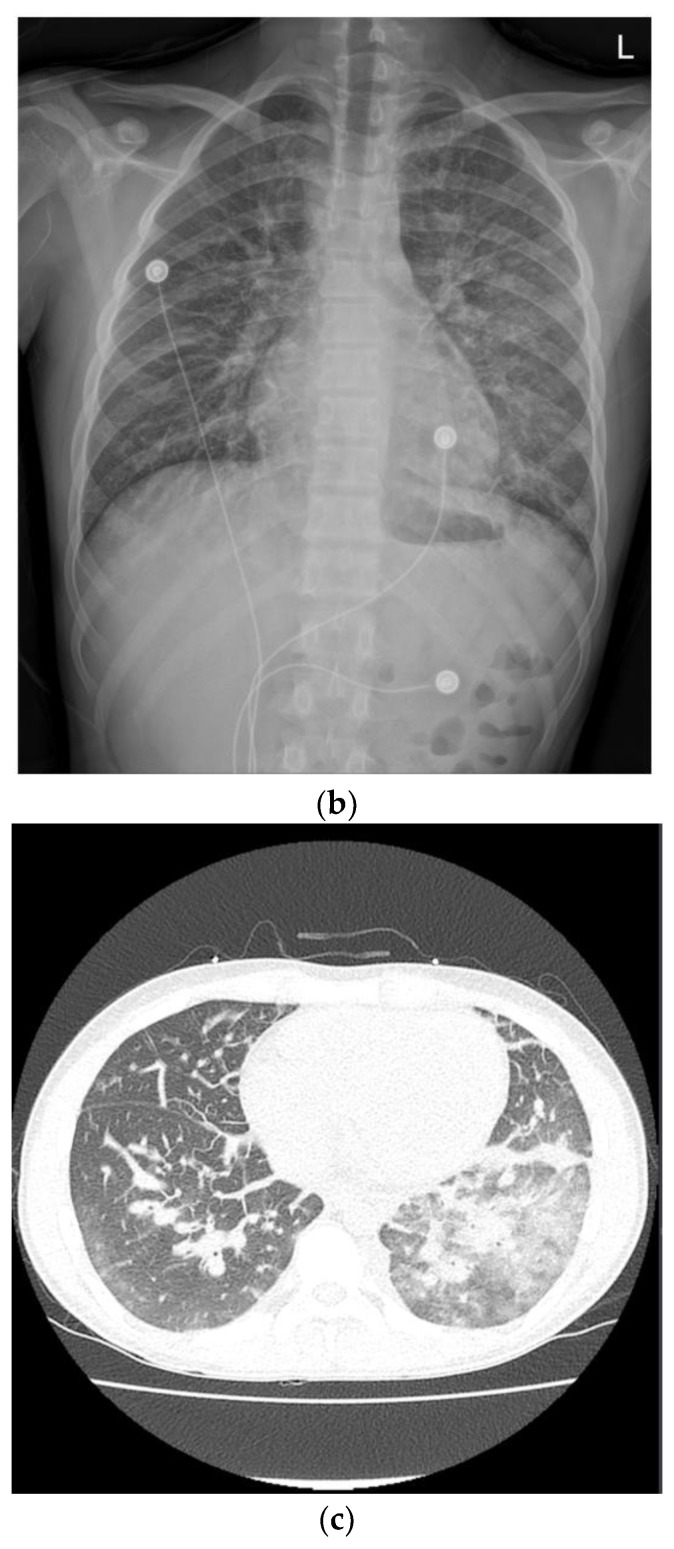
Compared with the chest radiography (a) at the time of admission, the chest radiography (b) and low-dose chest computed tomography (c) performed on the second day of hospitalization revealed pulmonary edema and cardiomegaly findings (b).
